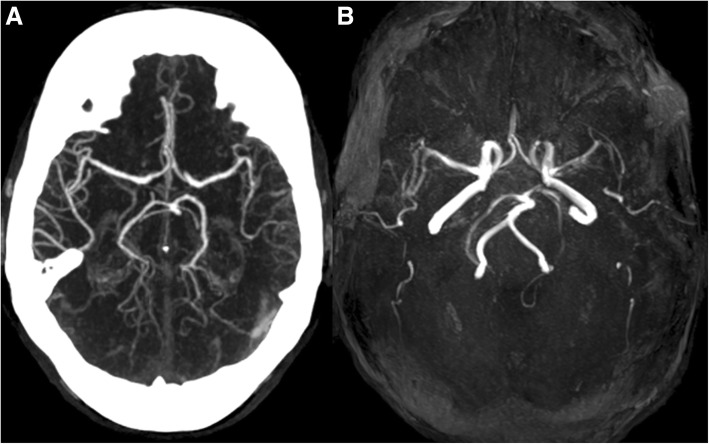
CT angiography (a) and MR-TOF angiography (b) did not reveal any evidence of intracranial arterial stenosis or occlusion
